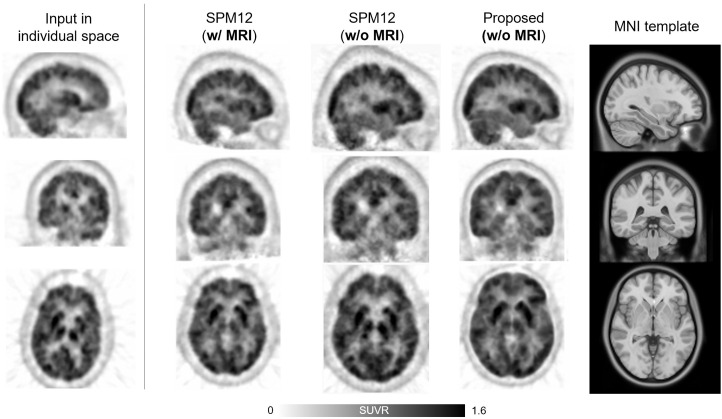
^18^F-FDG PET images of 67-y-old woman selected from an external dataset, exhibiting relatively small brain size, high noise level, and poor spatial resolution.
